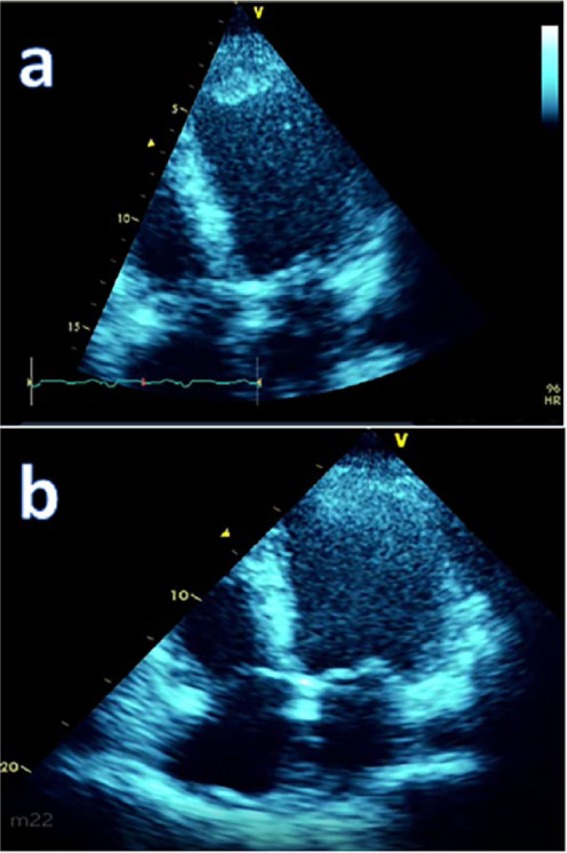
(a) The apical 25 mm × 15 mm left ventricular thrombus. (b) Complete dissolution of the preexisting apical left ventricular thrombus after adopting the antithrombotic strategy.
